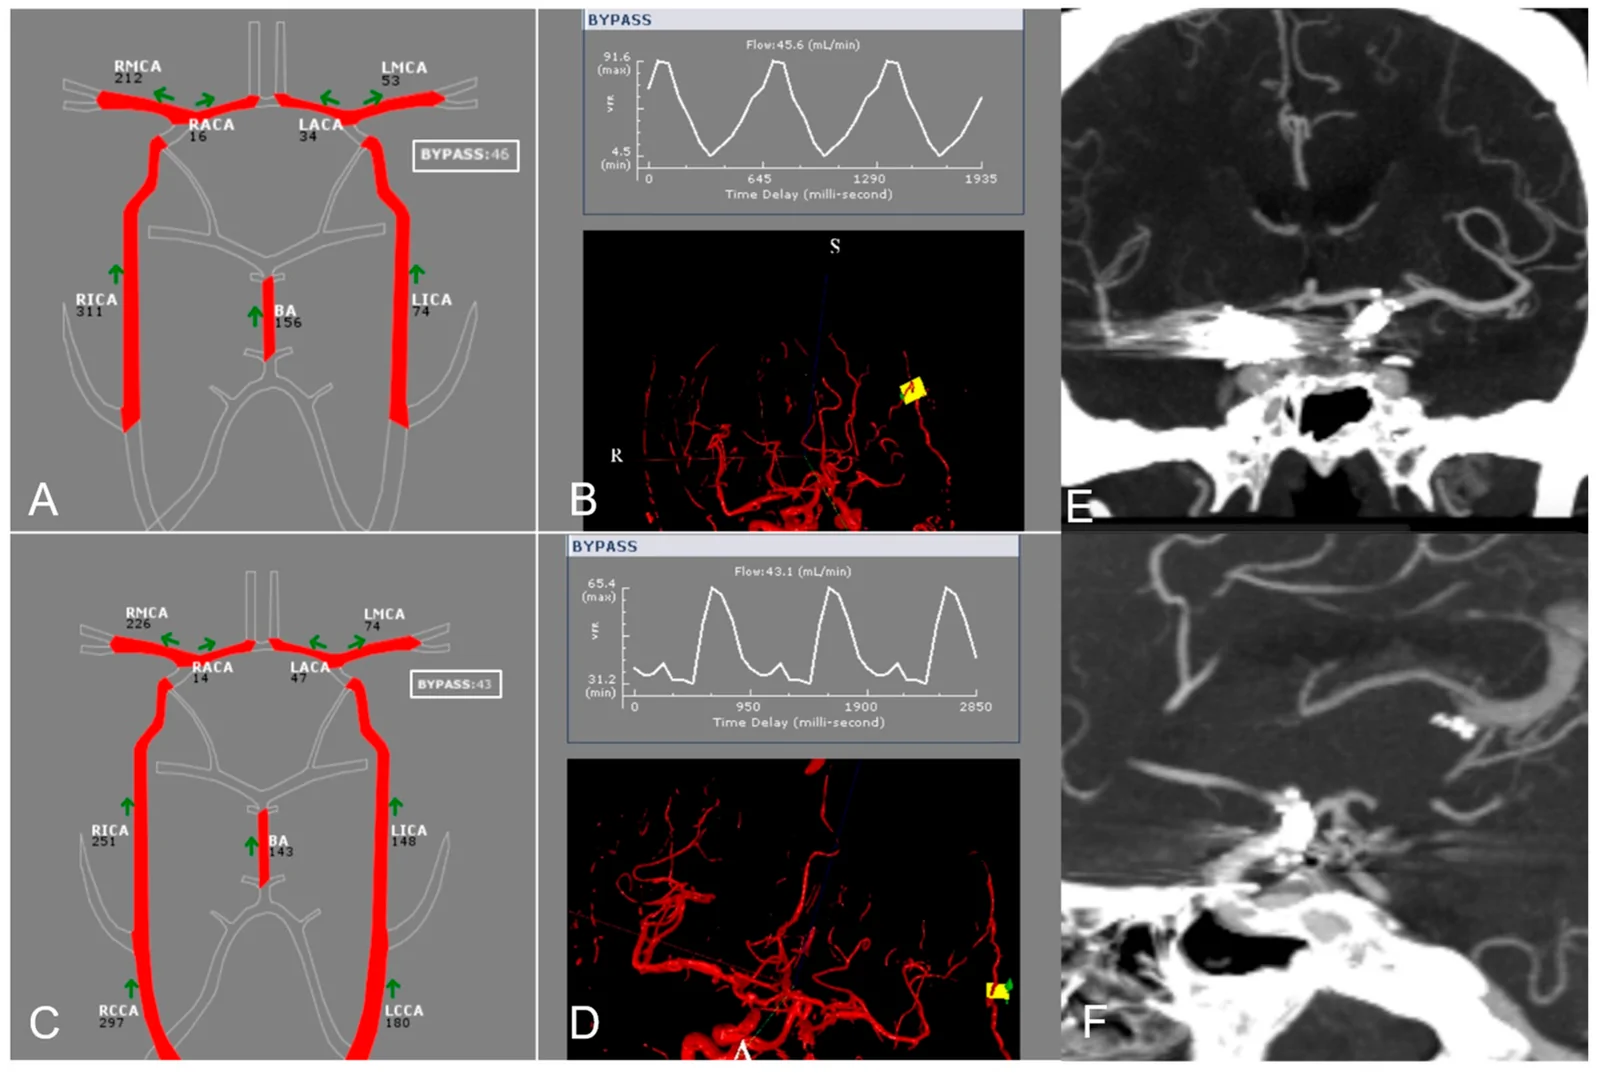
Postoperative qMRI NOVA^®^ demonstrated a flow of 53 mL/min in the MCA and 46 mL/min in the bypass (A,B). At the 7-day follow-up, NOVA^®^ showed stable bypass flow (46 vs. 43 mL/min) and a slight increase in MCA flow (53 vs. 74 mL/min) (C,D). The 4-year DE-CTA control showed complete exclusion of both aneurysms, patency of the FD and occlusion of the bypass, with restoration of physiological flow in the left MCA (E,F). RMCA: right middle cerebral artery; LMCA: left middle cerebral artery; RACA: right anterior cerebral artery; LACA: left anterior cerebral artery; BA: basilar artery; RICA: right internal carotid artery; LICA: left internal carotid artery; RCCA: right common carotid artery; LCCA: left common carotid artery.
